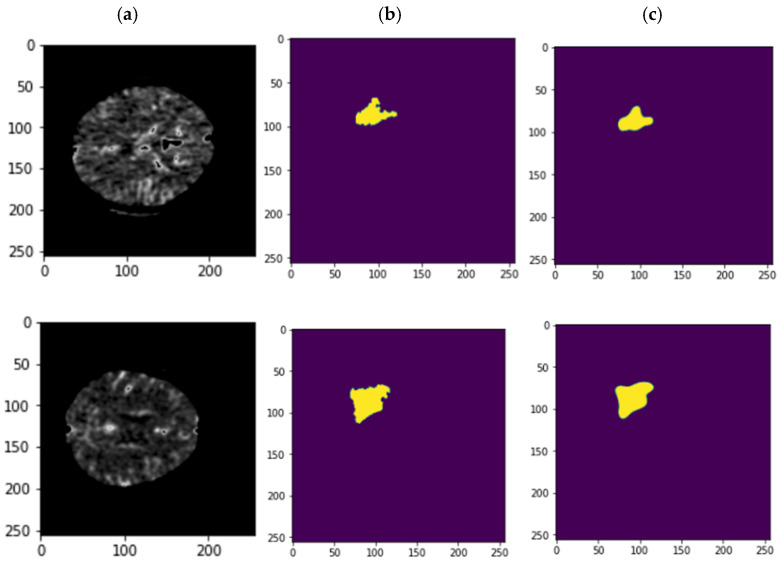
Comparison CT image (a), corresponding mask (b), and performance of the proposed model in diagnosing ischemic stroke (c).
